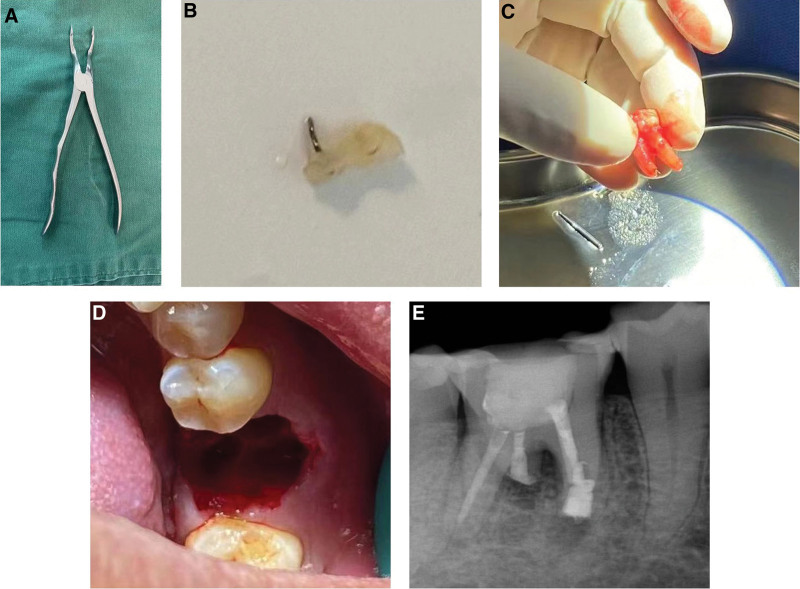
Surgical procedure: (A) atraumatic dental forceps were used to extract the tooth; (B) apical fragment with separated file is removed; (C) extracted tooth is kept wet with saline; (D) extraction socket is fresh and intact after removal of granulation; (E) immediate post-operative periapical radiograph revealed that the tooth was in place.
